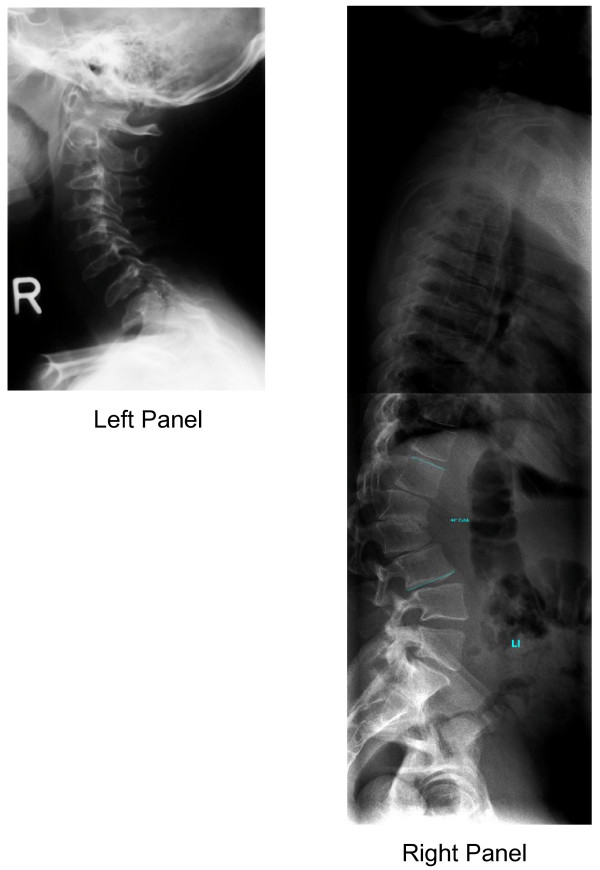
Spine in Attenuated MPS I. Left Panel: Cervical spine X-ray of an adolescent with attenuated MPS I showing ossification disturbance in the anterior part of column resulting in oval shaped vertebral bodies with anterior beaking and hypoplastic dens axis. Photo courtesy of Bianca Link. Right Panel: Complete spine X-ray in an adult patient with attenuated MPS I showing nearly normal shaped vertebral bodies and gibbus deformity at the thoracolumbar junction. Note that lateral X-rays of the thoracolumbar spine can also reveal anterior beaking. Photo courtesy of Bianca Link.
